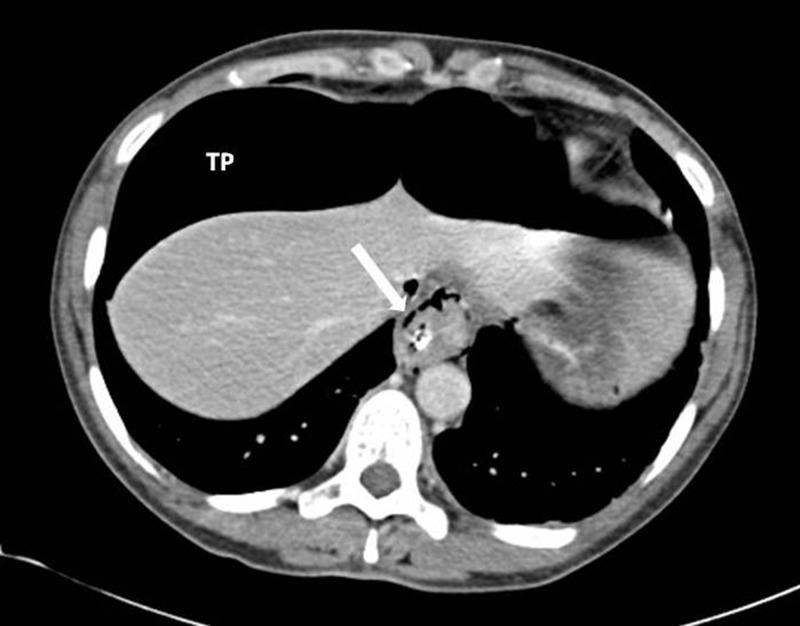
Computed tomography showing a small amount of gas in the soft tissues
